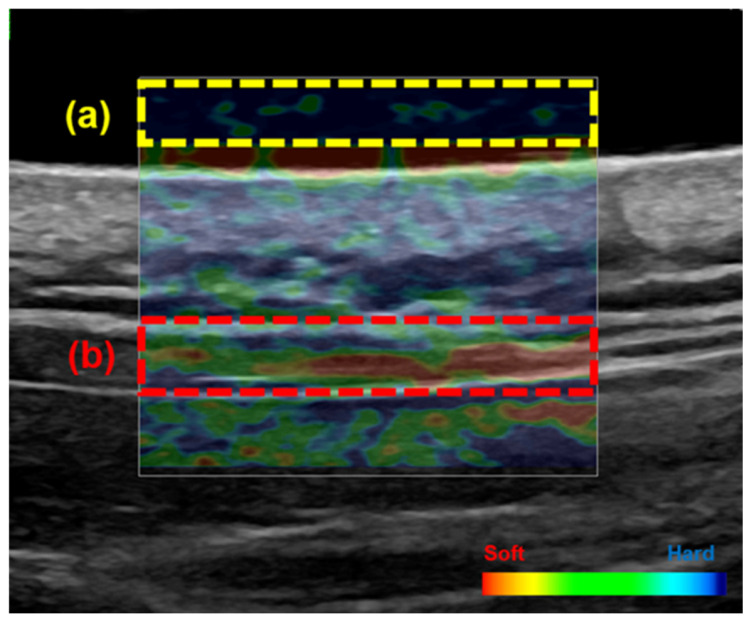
Ultrasound strain elastography image of the upper trapezius muscle. Regions of interest (ROIs) were defined for the acoustic coupler gel (a) and the upper trapezius muscle (b). Areas displayed in red represent softer tissue, whereas areas shown in blue indicate stiffer tissue. Muscle elasticity was quantified by calculating the strain ratio between the trapezius muscle and a gel pad, using a scale ranging from 0 (softest) to 6 (stiffest). A higher strain ratio indicated greater muscle stiffness.
